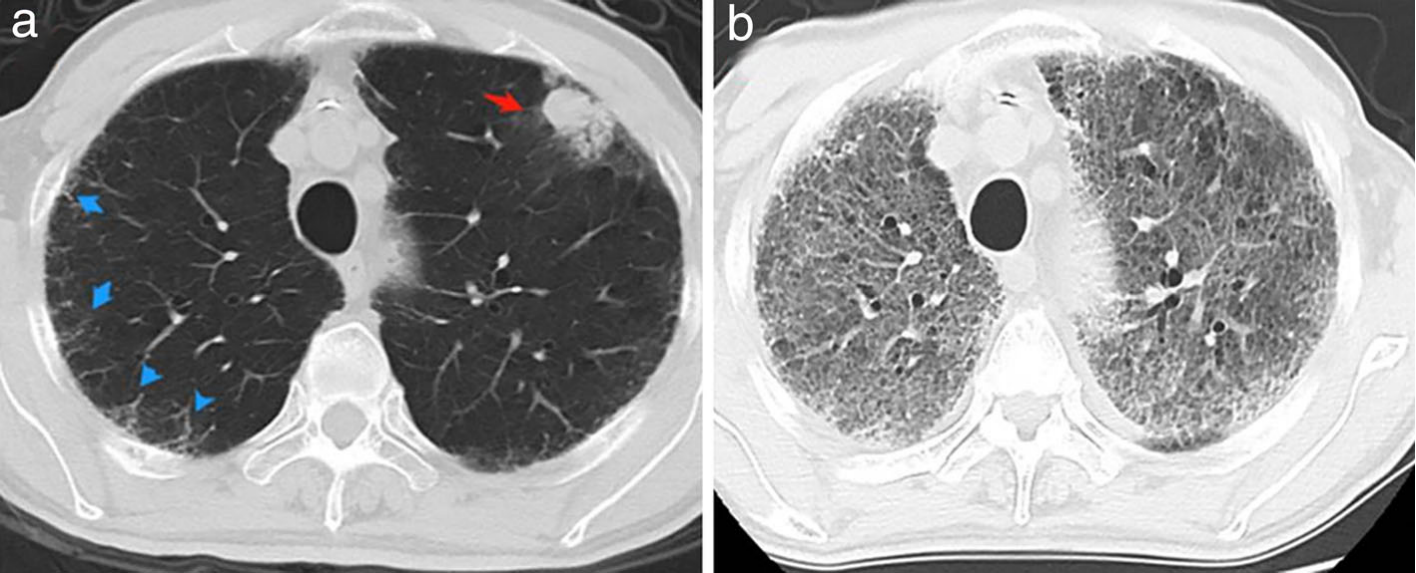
Chest CT before and after osimertinib treatment. (a) A round space‐occupying lesion with surrounding ground‐glass opacity was seen (indicated by red arrow) before osimertinib treatment. (b) A complete response after osimertinib treatment was achieved although severe interstitial lung disease was visible.
